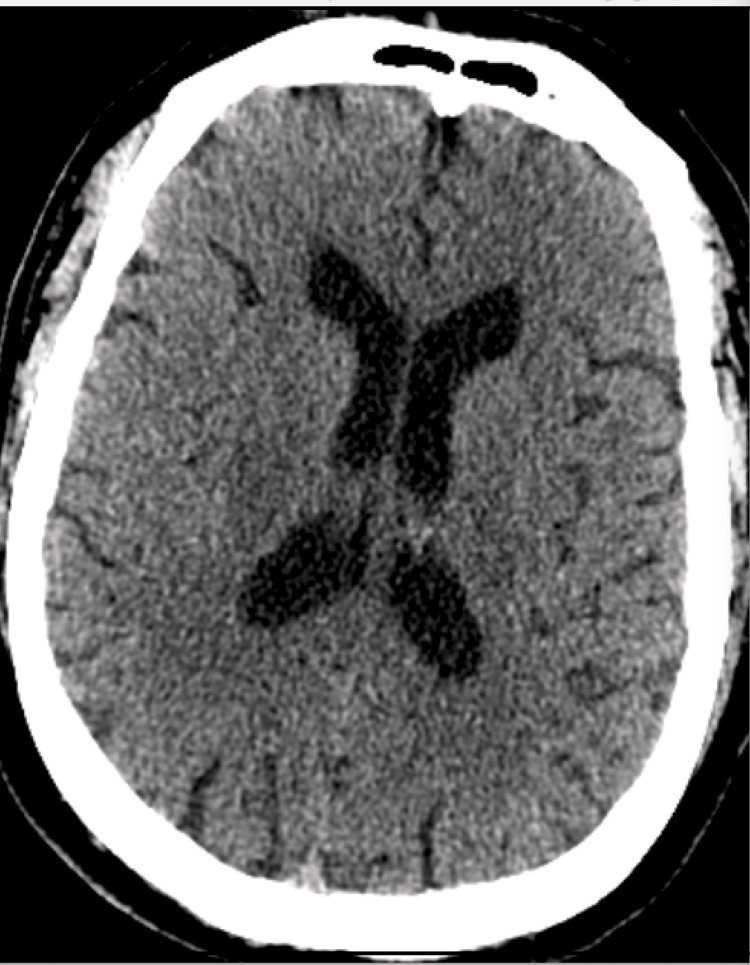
CT of the brain is unremarkable
